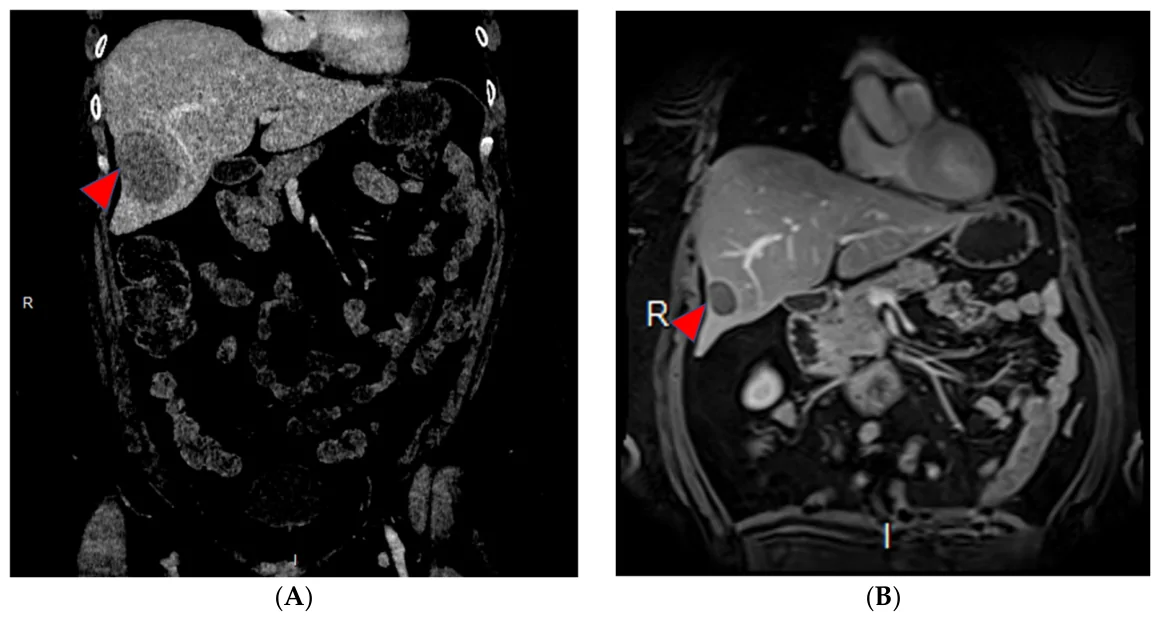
(A,B) Institutional review board approval was not required for this de-identified patient case. Patient with a metatstatic neuroendocrine tumor to segment 6 of the liver treated with histotripsy. (A) Metastatic neuroendocrine tumor in segment 6 (arrow) pre-histotripsy, coronal reconstruction. (B) Ten-month follow-up MRI with contrast showing interval decrease in size of the segment 6 mass (arrow), coronal reconstruction. Image orientation: R = right side of patient.
